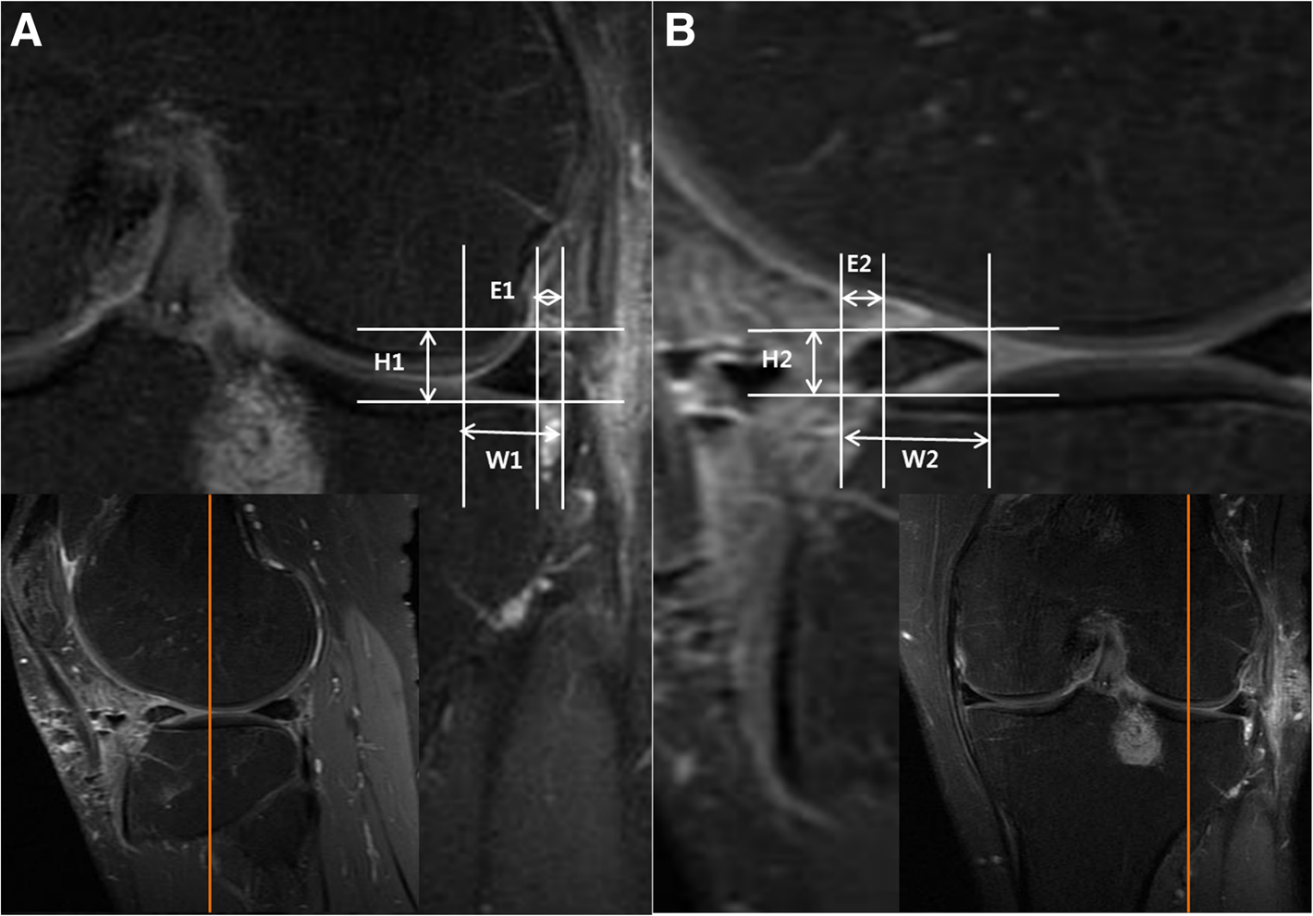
Measurements of graft extrusion or intrusion in the mid-sagittal plane and mid-coronal plane. From the mid-coronal knee MRI section (a), the radial extrusion (E1) was measured as the distance between the outer edge of the articular cartilage of the tibial plateau and the outer edge of the allograft. Also, the height (H1) and the width (W1) of the graft were measured. The radial relative percentage of extrusion (RPEr) was defined as the percentage of the width of extrusion relative to the width of the entire allograft (E1/W1 × 100). Similarly, the anterior relative percentage of extrusion (RPEa) was calculated after measuring the anterior extrusion (E2) and graft width (W2) from the mid-sagittal section (b). Graft height in sagittal section (H2) was also measured. Extrusion was expressed as a positive value and intrusion as a negative value
